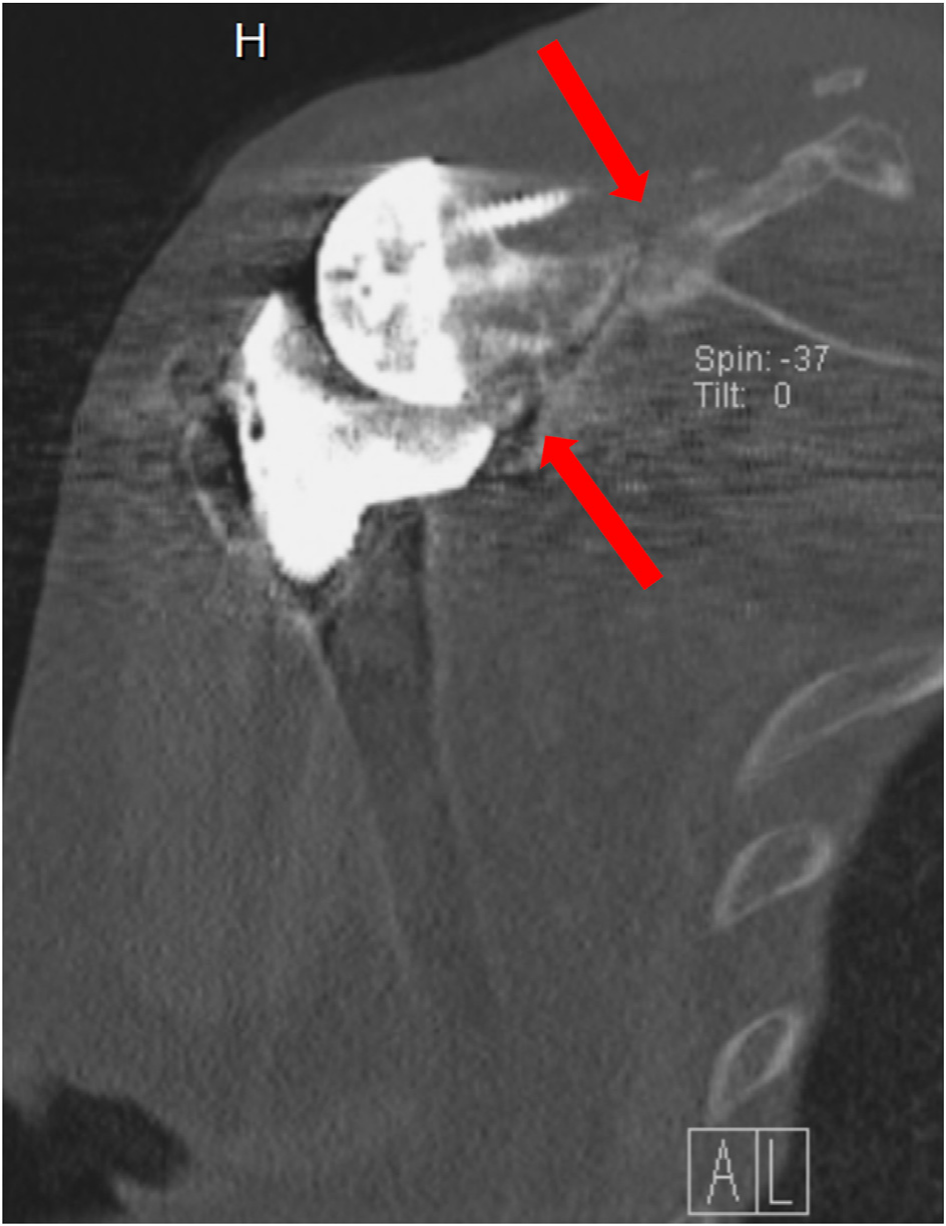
CT scan demonstrating a fracture inferior to the glenoid component. *CT*, computed tomography.
